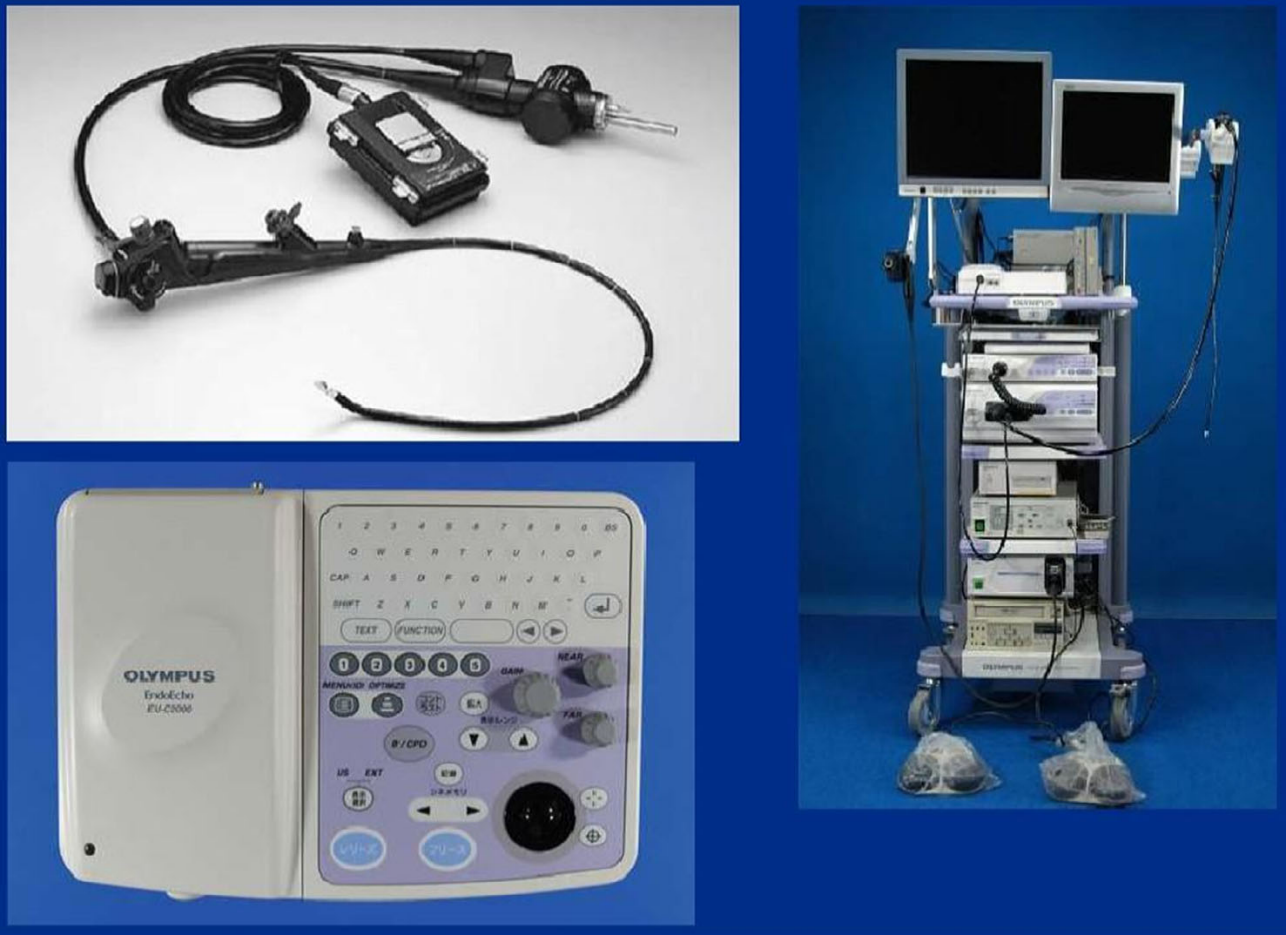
Ultrasonic probe preparation. Olympus endoscopic ultrasonography.
